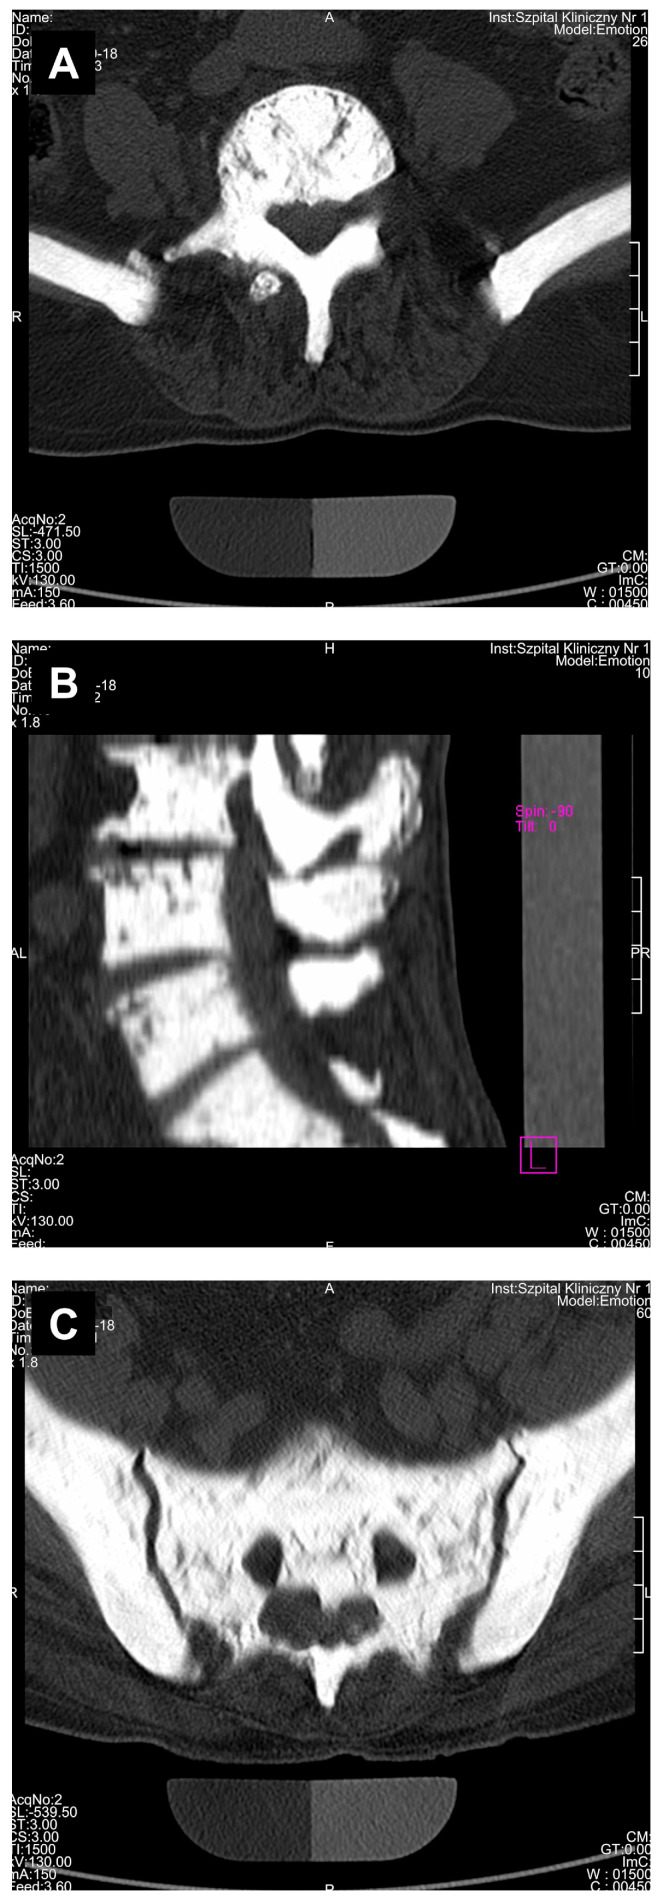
Osteosclerosis and marble view of the lumbar vertebrae (A,B), and pelvic (A,C) and sacral bones (C) in 80-year-old patient suffering from osteopetrosis visible on cross-sectional computed tomography reconstruction.
